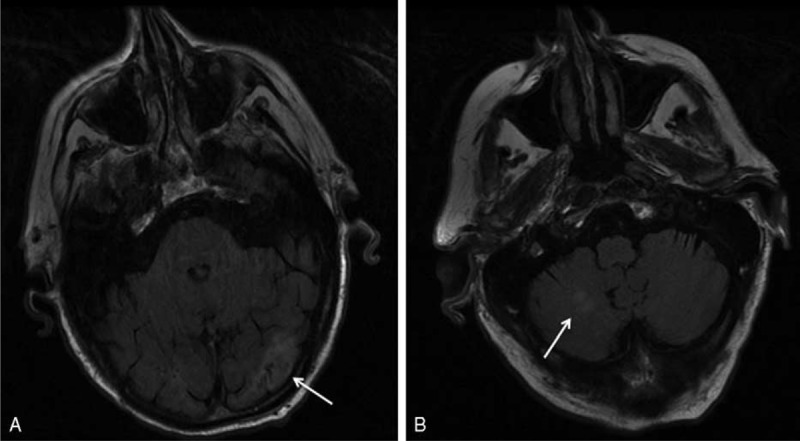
Cranial MRI. Left occipital (A) and right cerebellar (B) ischemic lesions.
